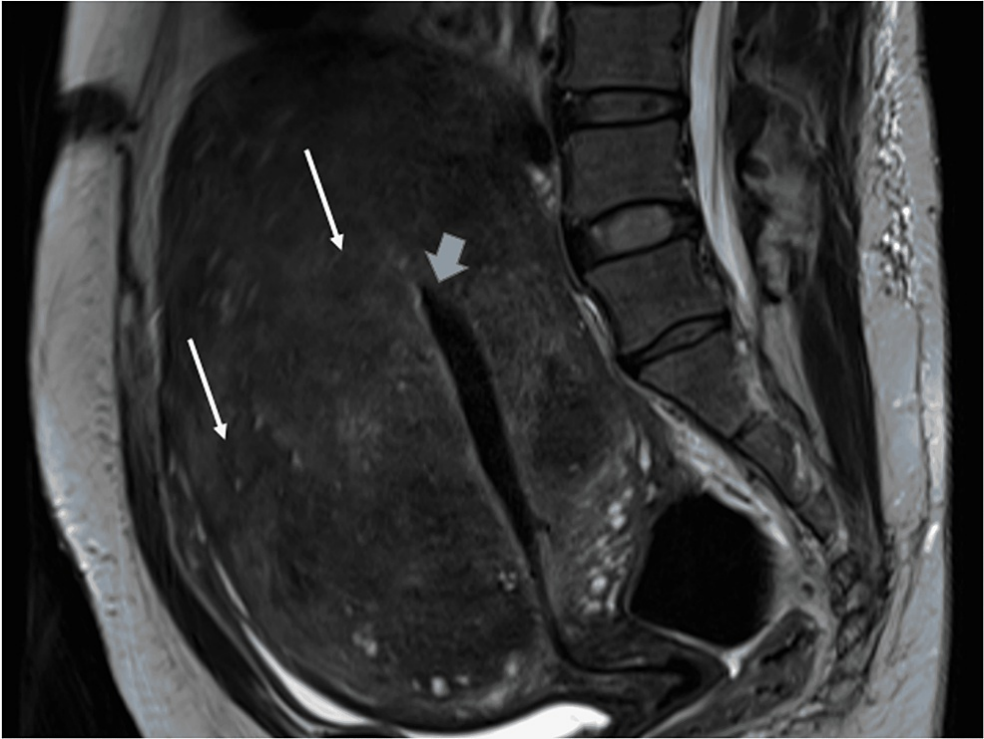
Pelvic MRI Sagittal section of MRI pelvis showing enlarged uterus with multiple small cysts within the myometrium (white arrows). The endometrial cavity is highlighted by the blue arrow, which shows thickened endometrium.
